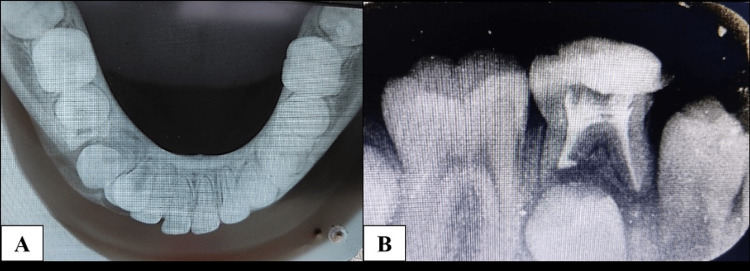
Occlusal and IOPA radiographs: (A) occlusal radiograph showing reduced buccal and lingual cortical plate expansion and (B) IOPA indicating pulpectomy with 85 (radiograph taken after obturation and before final restoration) and erupting the first premolar (44). IOPA, intraoral periapical
